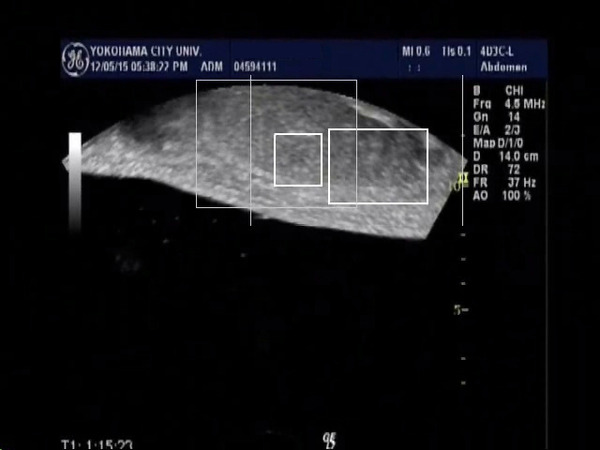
Tumor of the monitor ultrasonography in breath in position
